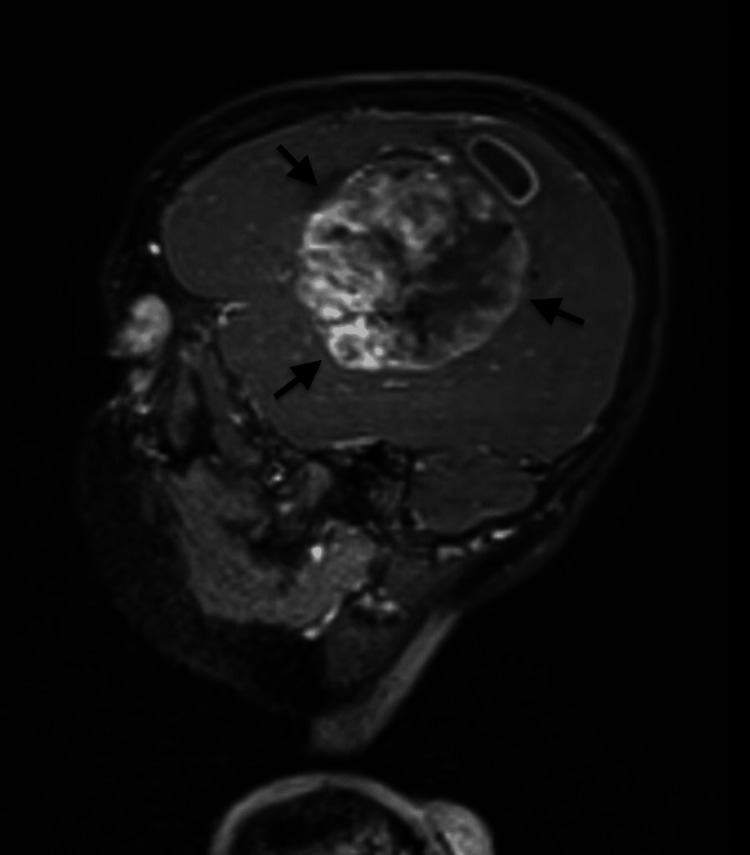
Preoperative magnetic resonance imaging scan in the T1 sagittal view Arrows are pointing toward the tumor.
